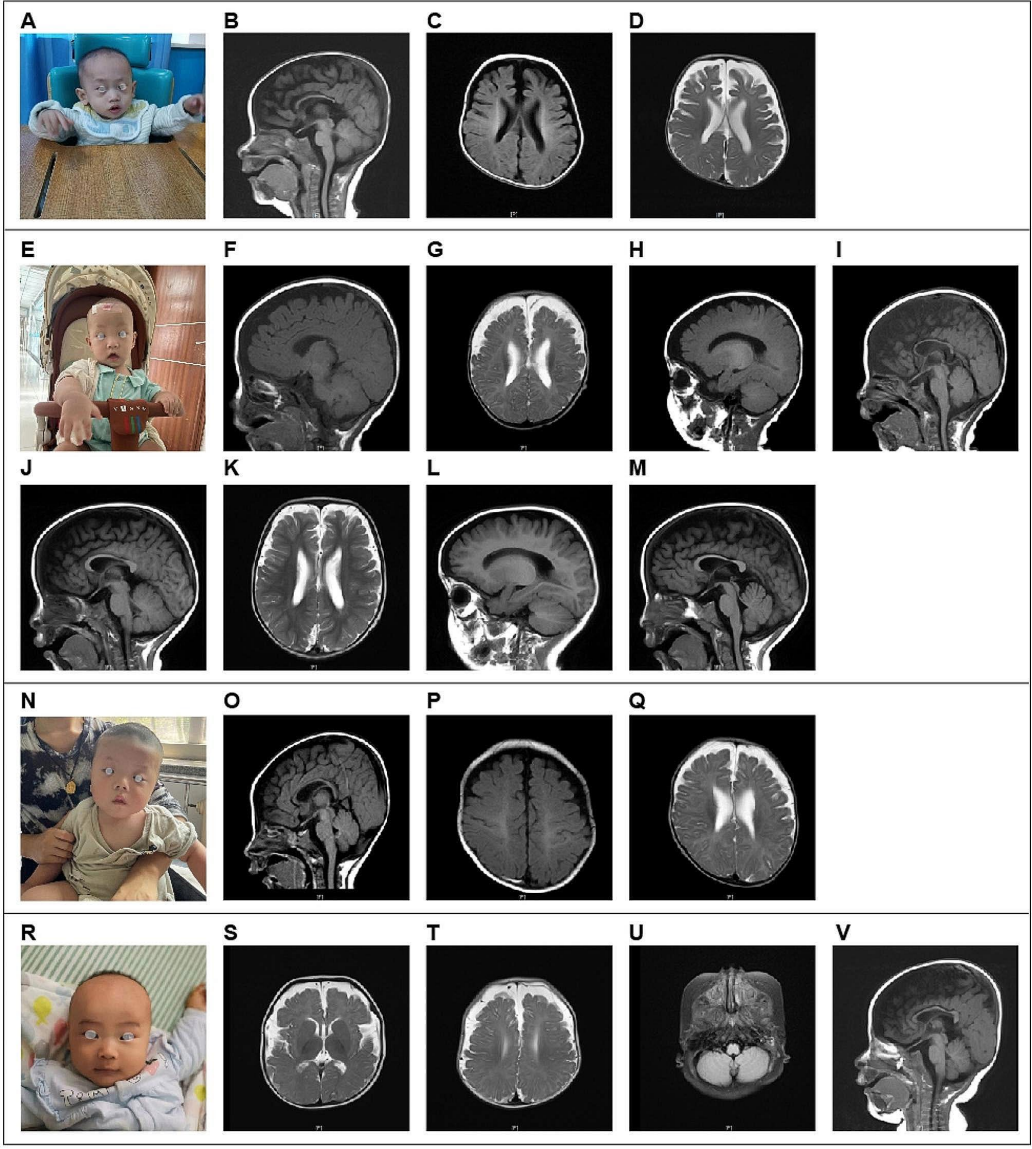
MRI indications in cerebral magnetic resonance imaging for the four cases. A–D: Case 1 had delayed myelination, widening of the bilateral ventricles, and dysplasia of the corpus callosum. E–I: (4 months and 9 days): Case 2 possessed a smaller brain mass, exhibited widening of the bilateral ventricles, and incomplete closure of the transparent compartments; the cerebral sulcus was slightly wider, the bifrontotemporal extracerebral space was significantly wider, and the corpus callosum was narrow; and a long T2 signal occurred in the bilateral mastoid; J–M: (1 year and 4 months): In Case 2, a reduction in the widening of the bifrontotemporal extraencephalic space and an increased in the widening of the bilateral ventricles was noted. N–Q: Case 3 had slightly delayed myelination, widening of the bifrontotemporal extracerebral space, irregular widening of the bilateral ventricles, and slightly thinner corpus callosum. R–V: Case 4 had a smaller frontal lobe volume, an old bleeding lesion in the left occipital lobe, a softened lesion with hemosiderosis, low signal in the right cerebellar hemisphere, significant widening of the bilateral frontotemporal extracerebral space, and thin corpus callosum
